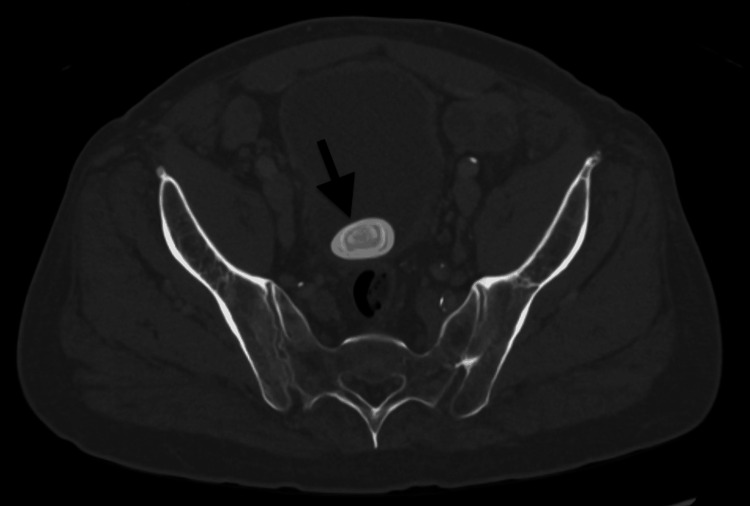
CT scan abdomen and pelvis with contrast (axial view) revealing a 3.5 cm bladder stone (arrow).
